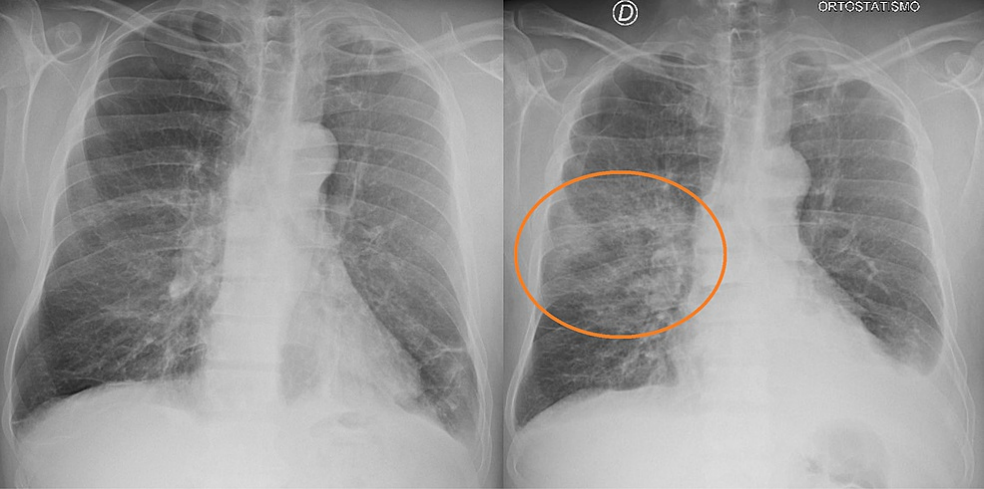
X-ray of the patient before (left) and after (right) radiotherapy X-ray image on the right shows radiation pneumonitis (orange circle)
